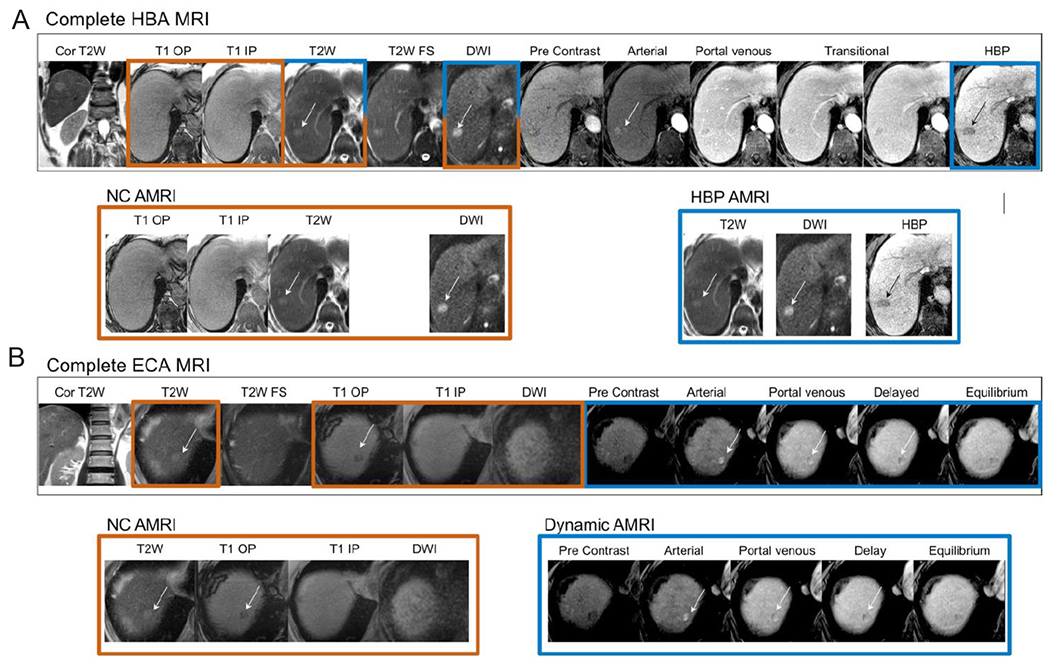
Complete MRI exams (A: HBA MRI; B: ECA) disaggregated into each of the three AMRI approaches (NC-AMRI, HBP AMRI and Dynamic AMRI). MRI: magnetic resonance imaging; NC AMRI: non-contrast abbreviated MRI; HBA: hepatobiliary agents; ECA: extracellular contrast agents; HBP: hepatobiliary phase
